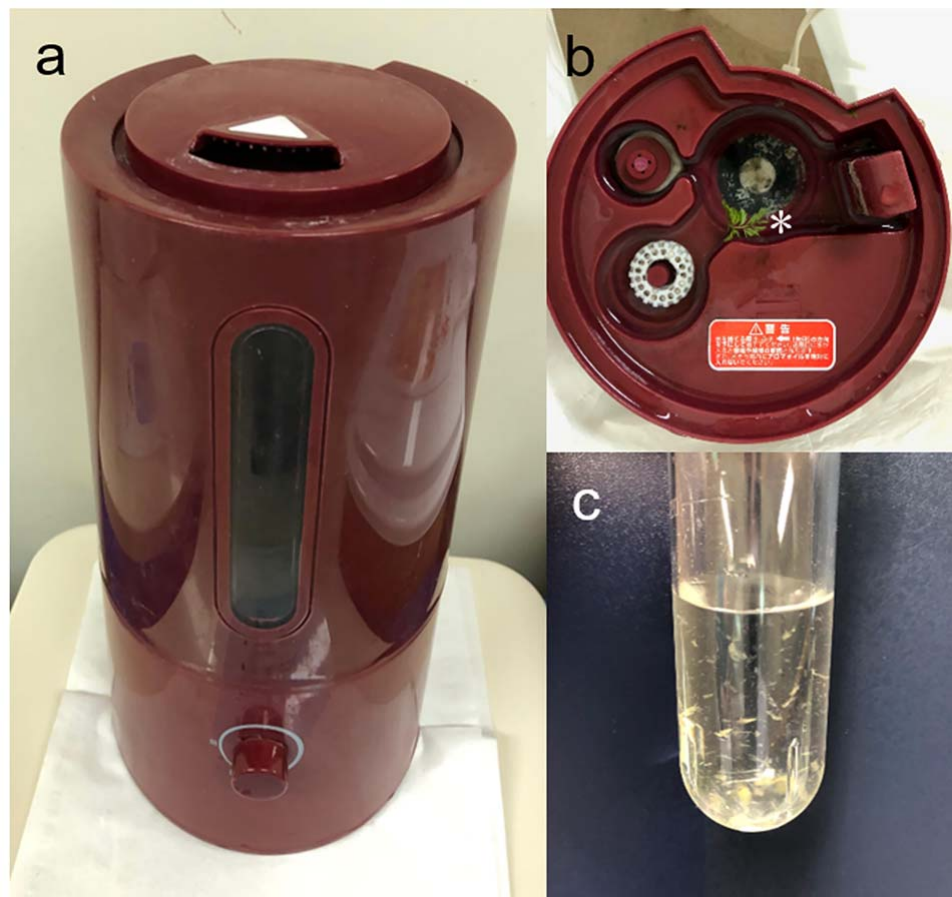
The ultrasonic humidifier used by the patient. (a) The humidifier. (b) The inside of the humidifier tank, which was not cleaned. The *asterisk* indicates some leaves that fell into the tank from a plant located next to the humidifier. (c) Turbid humidifier tank water containing dirty suspended materials.
